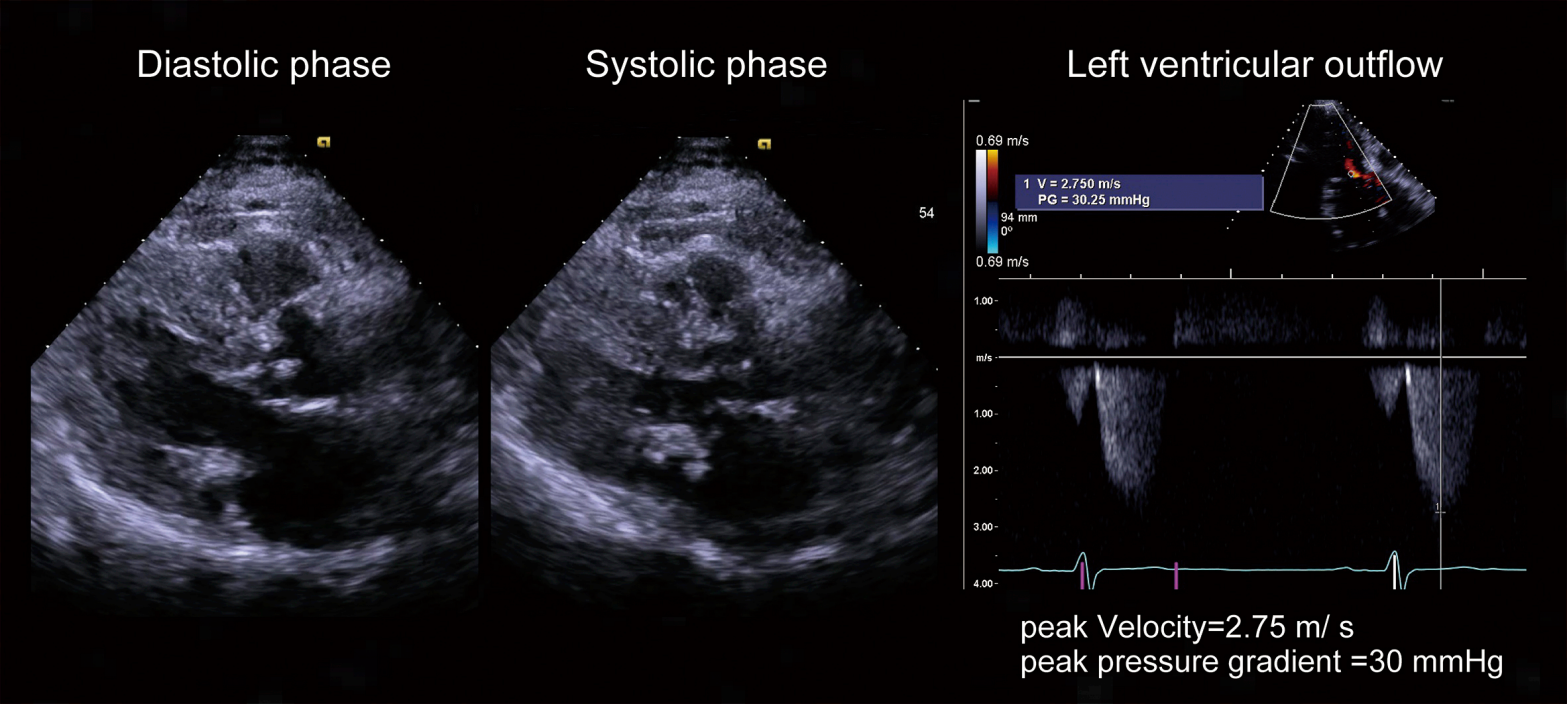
Echocardiography of the patient. The patient exhibited asymmetric septal hypertrophy; the interventricular septum measuring 16 mm and the left ventricular posterior wall measuring 9.1 mm (left and middle panel). Additionally, increased blood flow through the left ventricular outflow tract confirmed the diagnosis of obstructive hypertrophic cardiomyopathy (right panel).
